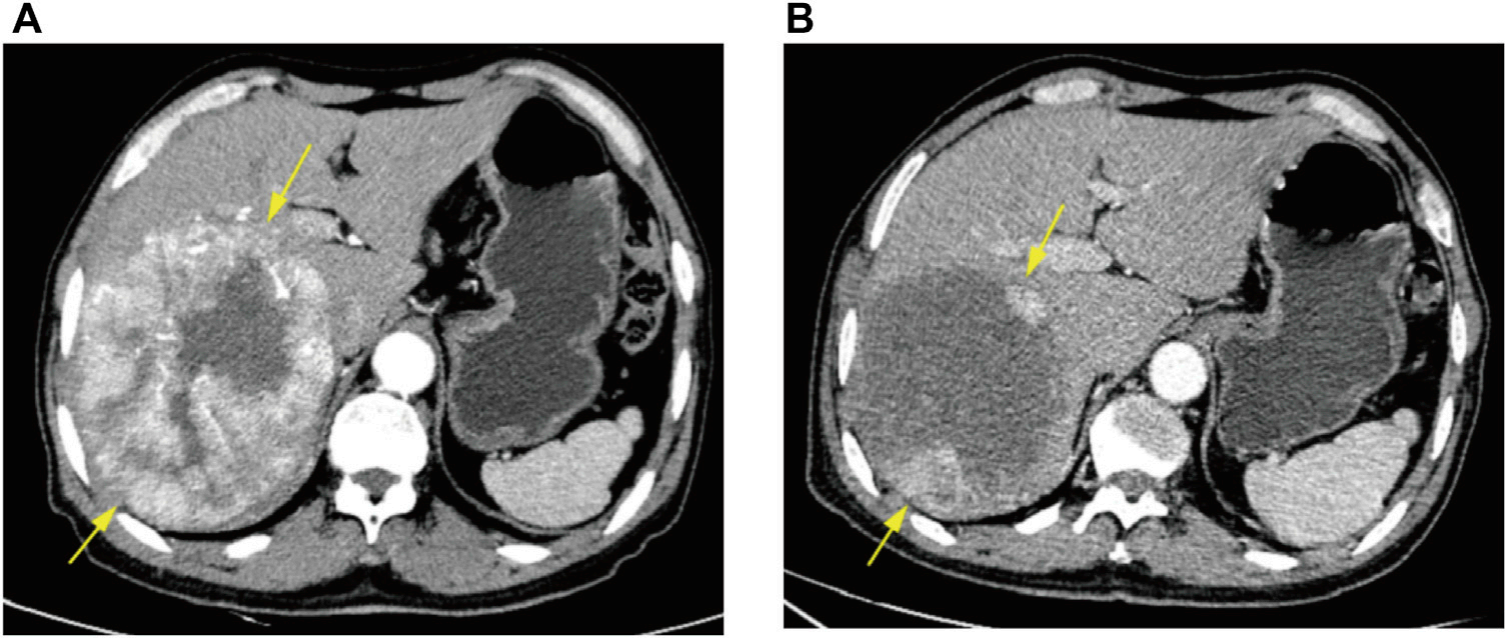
CT arterial phase images of a 66-year-old male patient before DEB-TACE and at 6 weeks postoperative re-examination. (A) Preoperative enhanced CT demonstrating massive HCC in the right lobe of liver. (B) Postoperative enhanced CT signifying nodular enhancement of the surviving tumor surrounding the wall of the original lesion area, with efficacy assessed as PR.
